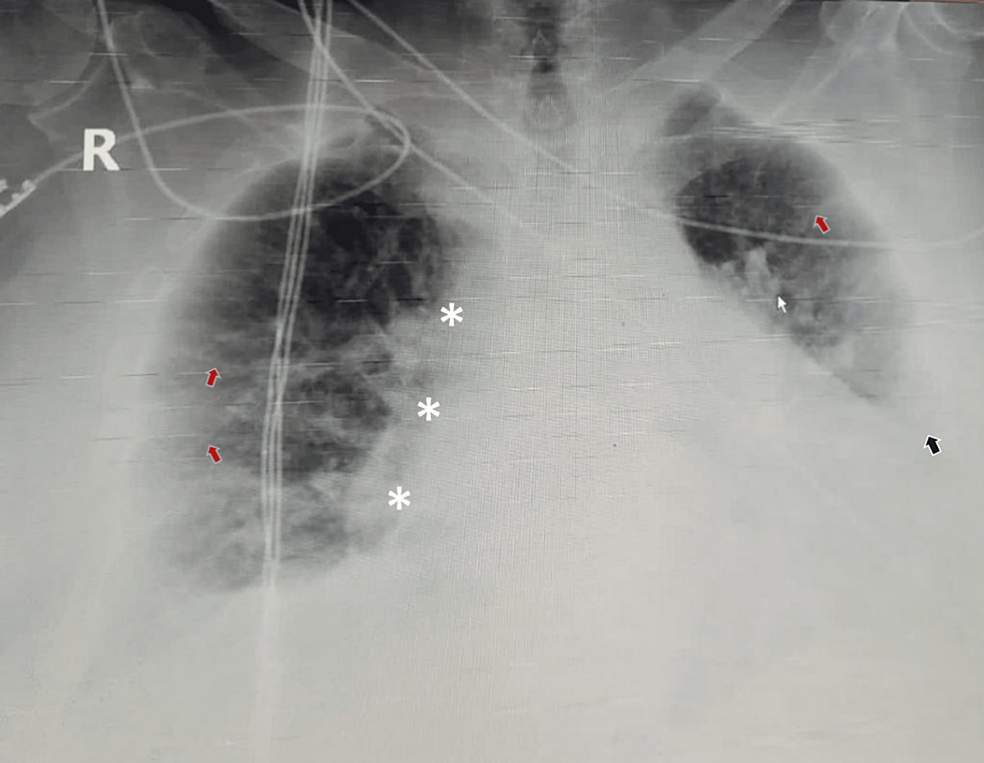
Chest x-ray of the patient shows bilateral infiltrations with diffuse linear increased interstitial markings throughout both lungs (red arrows), shaggy outline around the heart (*), and obliteration of left costo-phrenic angle (black arrow).
